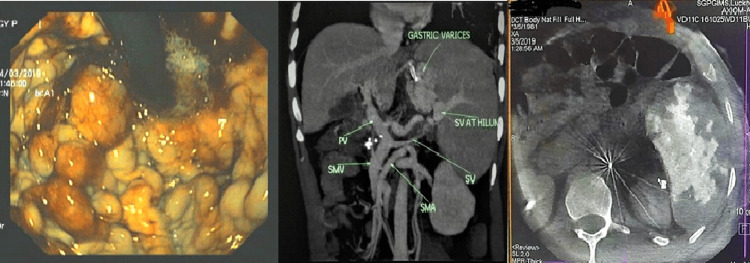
Endoscopic and imaging findings in case 1. The first image is an endoscopic picture showing multiple columns of large gastric varices (>10 mm each) with red-colored signs in the gastric fundus. The middle image is a coronal maximum intensity projection (MIP) CT reconstruction showing attenuated splenic vein (SV) and gastric varices with a patent portal vein. The third image is an intraprocedural cone beam CT, showing patchy enhancement of the spleen post-embolization.
